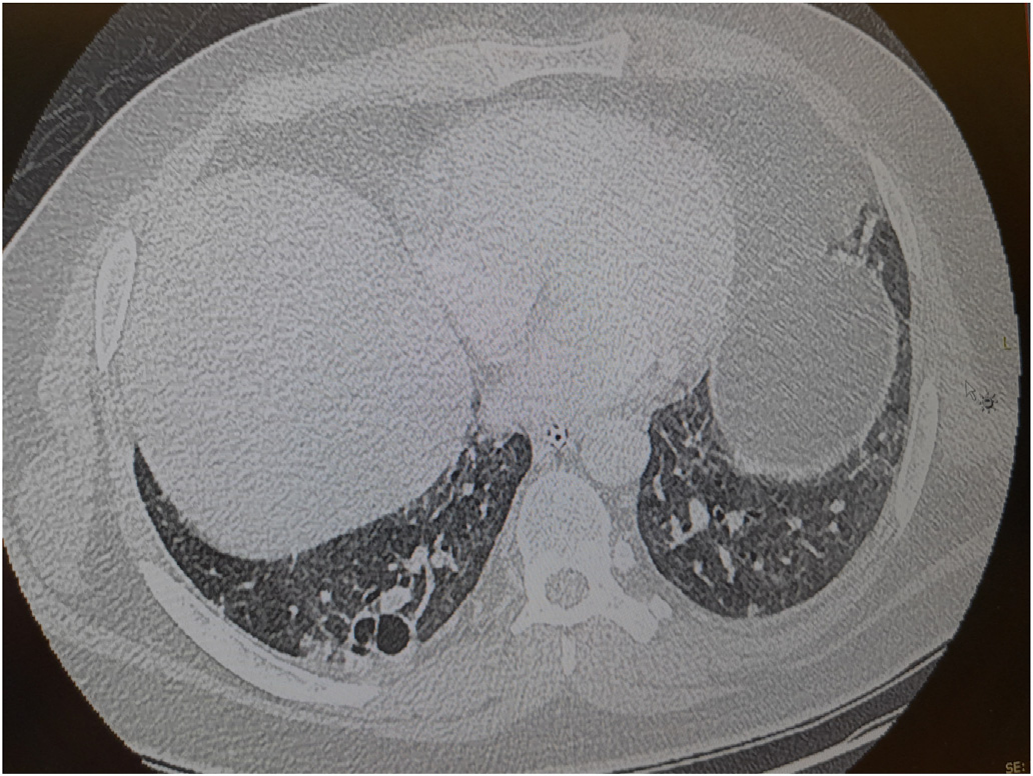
Chest computed tomography-scan performed on day 40 after onset of symptoms showed excavations in right lower lobe with residual ground glass opacities.
